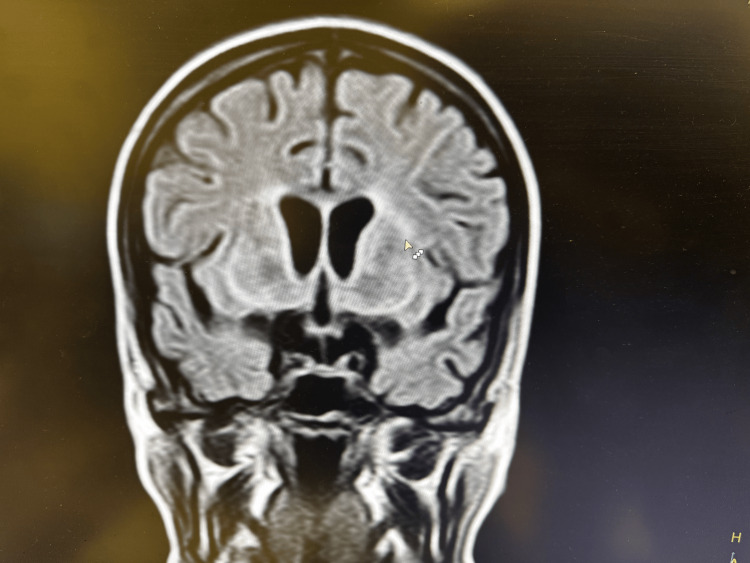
MRI of the brain showing perivasculitis as indicated by the small white arrow
